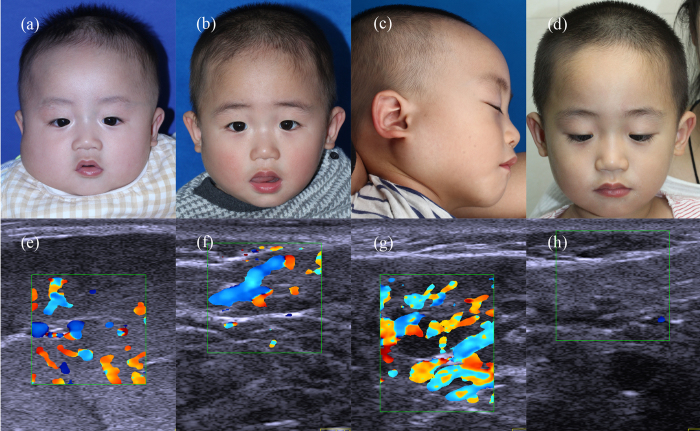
Another typical case involving partial regression. (a) Before treatment, a 4-month-old boy presented with a deep hemangioma in his right parotid region. (b) After 11 months of propranolol treatment, maximal reduction was achieved for more than three months. Propranolol was stopped gradually over 4 weeks. (c) One month later, progressive regrowth of the deep component of the hemangioma was observed. (d). No recurrence was observed 6 months after a second course of propranolol treatment for 7 months. (e,f,g,h) Corresponding color Doppler ultrasound images of (a,b,c and d).
